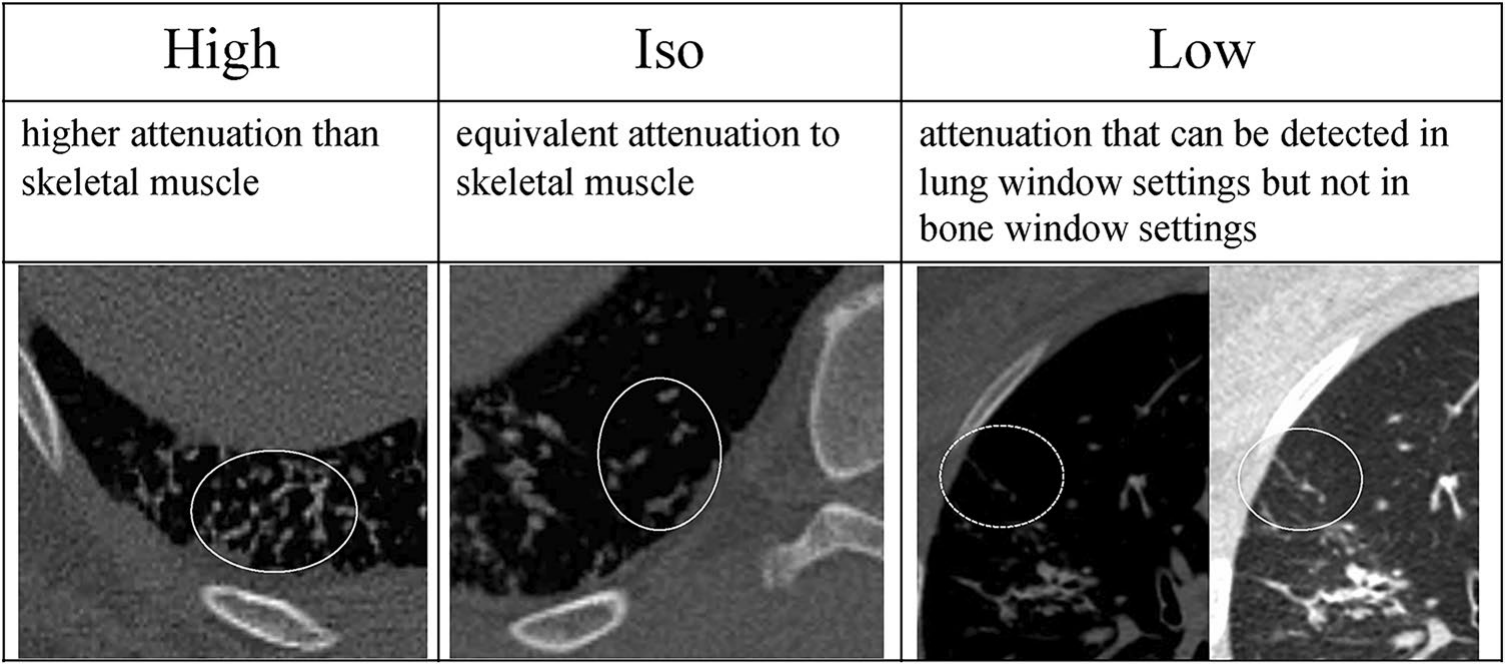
CT attenuation level at bone window settings (WW 2500HU, WL 500HU). After evaluating the presence of branching, linear, and round structures in lung window settings, the attenuation values of these structures were classified into three levels in bone window settings (WW 2500HU, WL 500HU): high, higher attenuation than skeletal muscle; iso, equivalent attenuation to skeletal muscle; and low, attenuation that can be detected in lung window settings but not in bone window settings (shown in the circle of each image). Figure 5 shows a representative case of “high”, and Fig. 6 shows the representative case of “iso”
